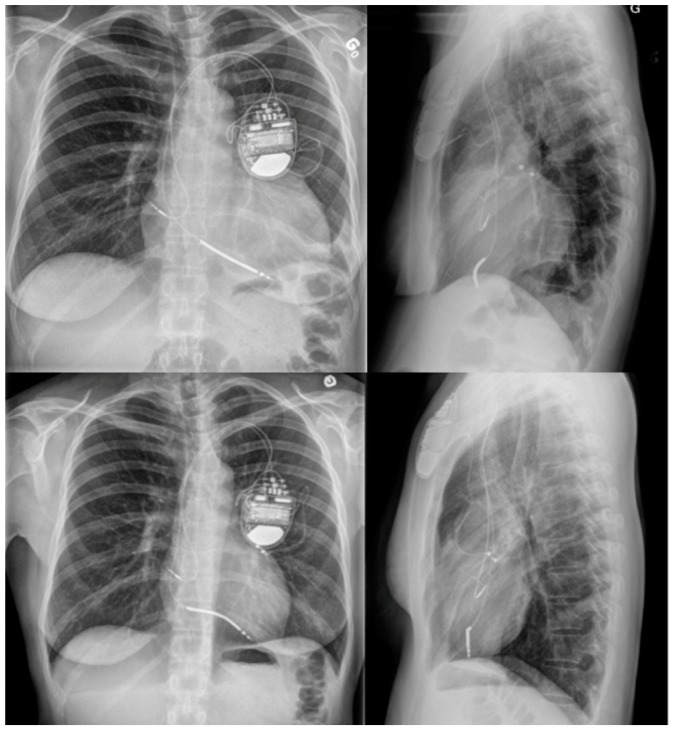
Chest X-rays imaging CRT leads position and increased cardio-thoracic index immediately after implantation (upper line). Remarkable normalization of cardio-thoracic index after 13-month follow-up (lower line).
